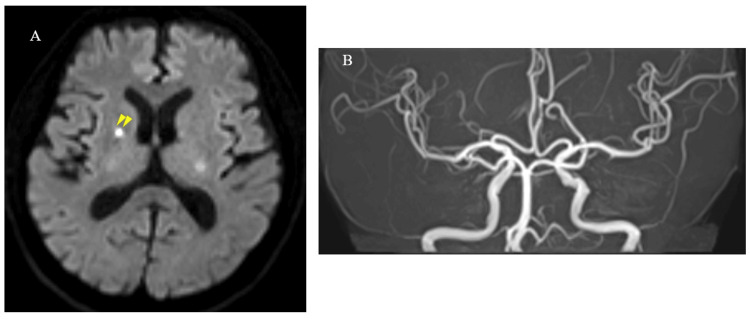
Brain MRI Findings at the Time of Stroke Recurrence (Day 43 of Hospitalization) (A) A new infarct was observed in the right putamen (arrowheads). (B) Intracranial magnetic resonance angiography (MRA) revealed no evidence of stenosis or occlusion in the major cerebral arteries.
